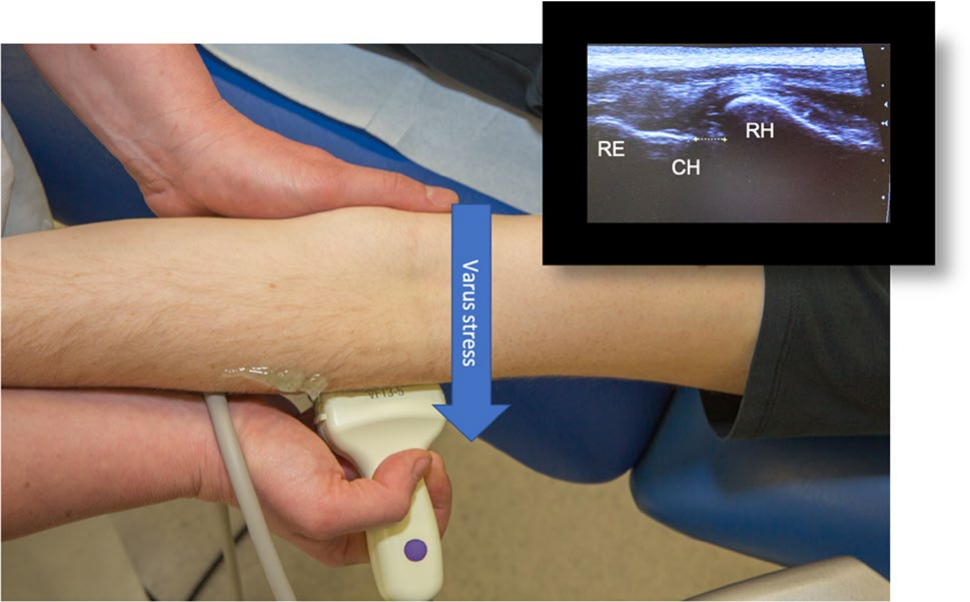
Patient in supine position, ultrasound of the lateral left elbow (landmarks: radial epicondyle (RE), capitulum humeri (CH), radial head (RH)) with focus on the joint line. Varus stress testing in full extension measuring the joint space between the CR and the RH
